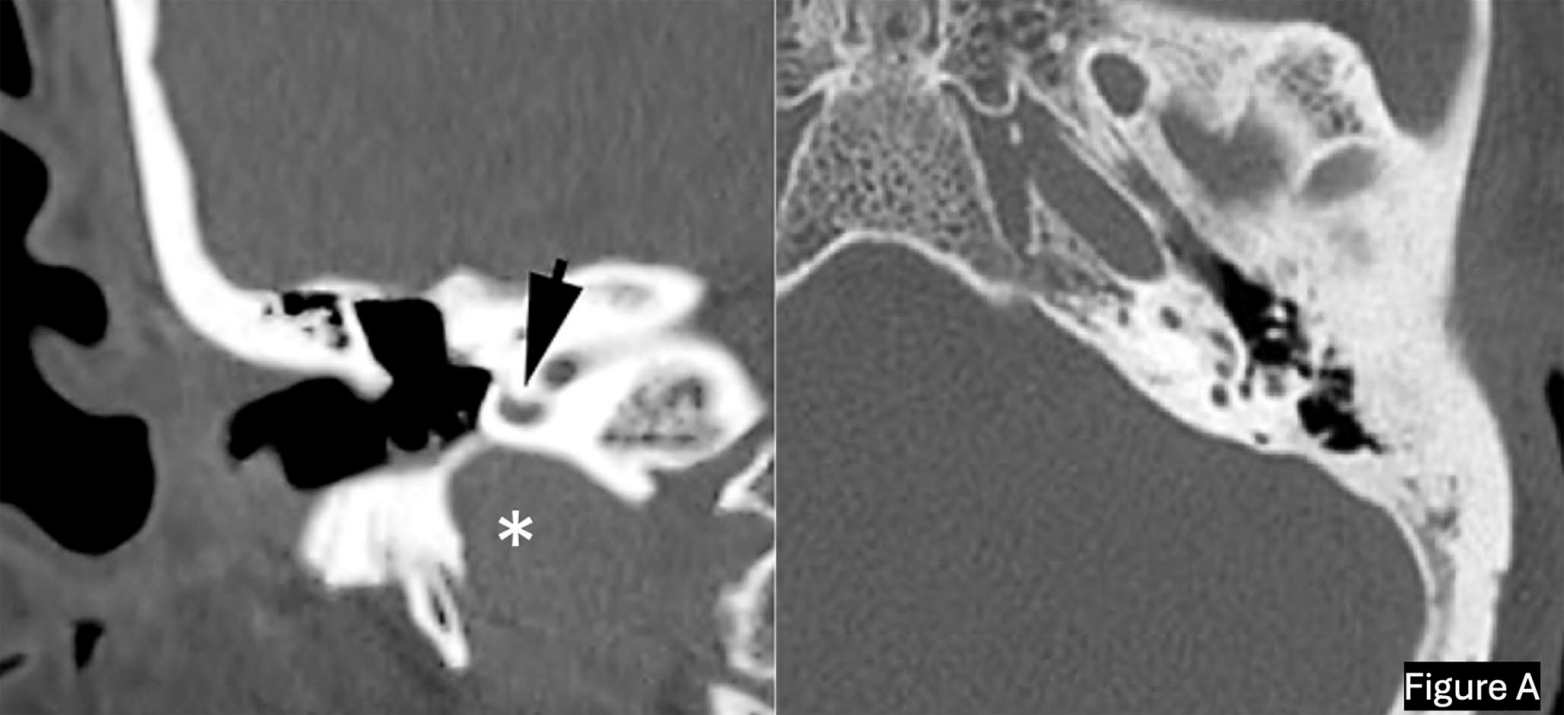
In the left pre-operative high-resolution CT (HRCT) scan is visible an example of a high jugular bulb (white asterix) in direct contact with the basal turn of the cochlea (black arrow) in a patient with a history of tympanoplasty and a current indication for CI; on the right HRCT scan, left temporal bone ossification of the round window, basal and apical turn of the cochlea secondary to otosclerosis
